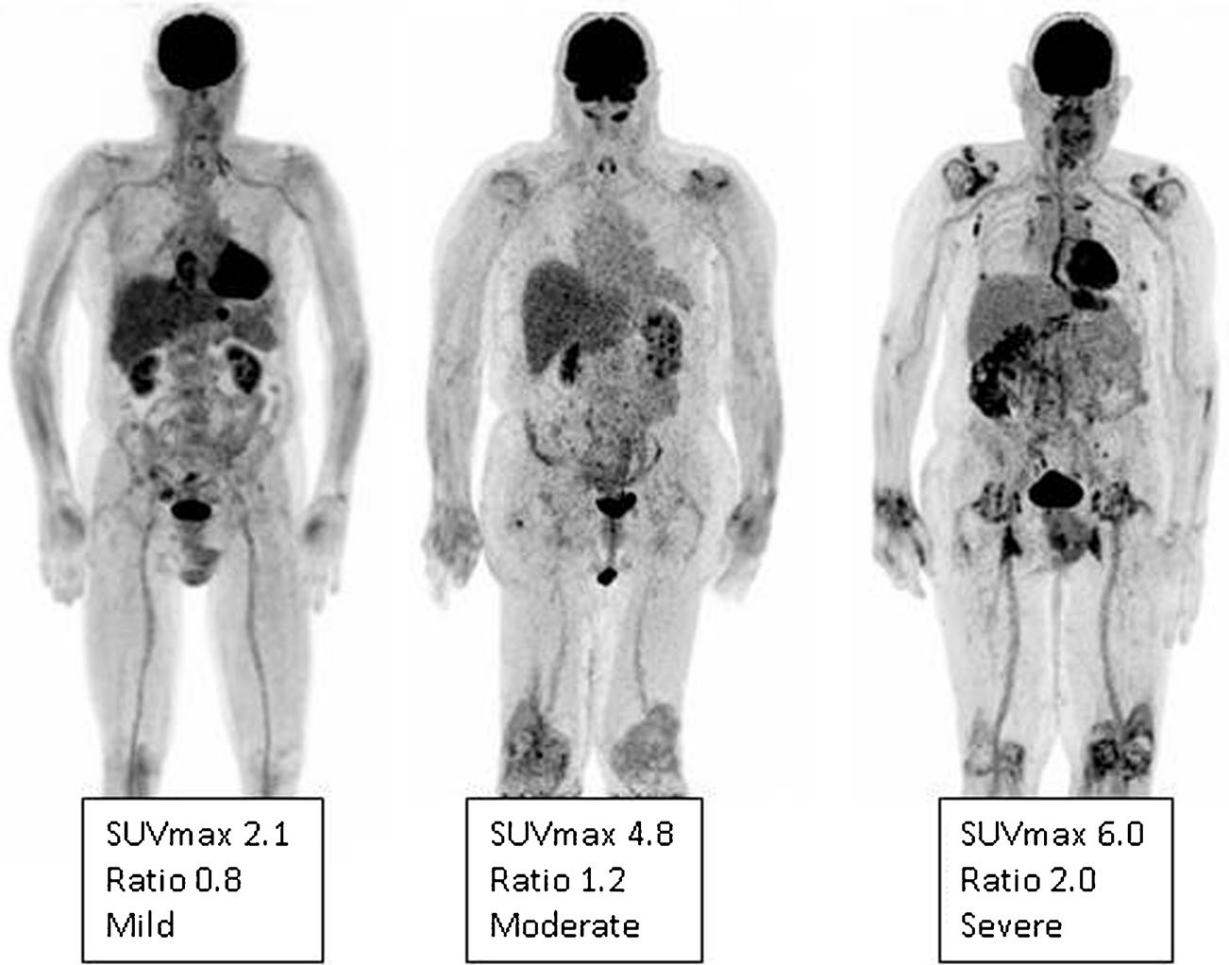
FDG-PET. Low (grade 1), intermediate (grade 2), and high (grade 3) FDG uptake patterns of the large joint regions in PMR patients, including SUV_max_ of the shoulders. Ratio is defined as average SUV_max_ in the shoulders divided by the liver region. The total number and intensity of affected joints is the highest for the right-positioned patient
